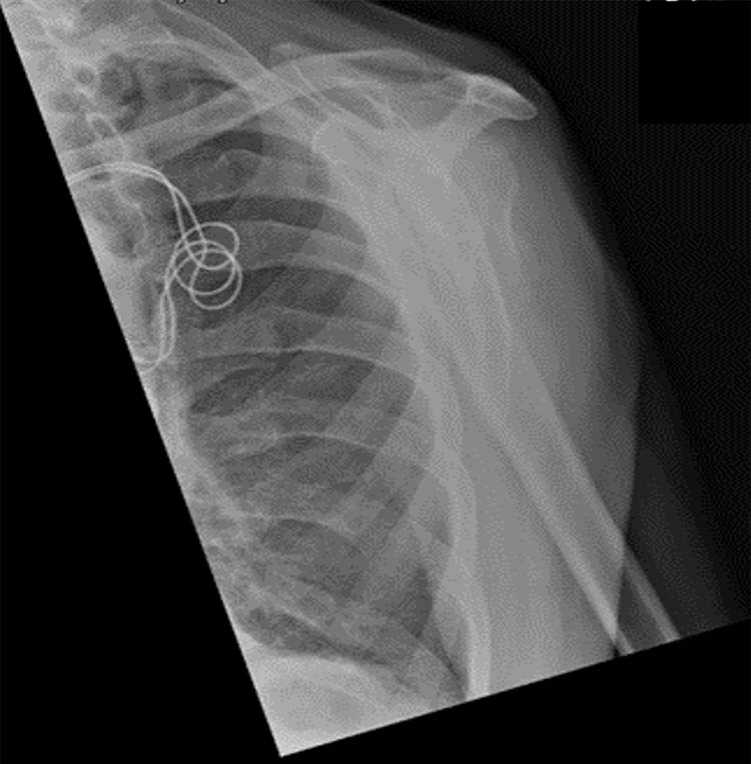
Lateral scapula (Y) view X-ray taken 6 weeks post-injury showing radiological healing of the fracture.
